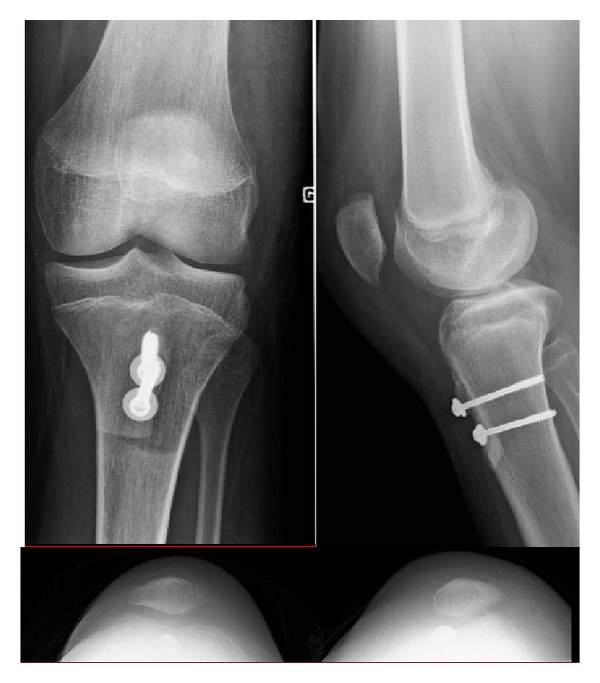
Postoperative radiograph of the knee after medial tibial tubercle transfer and vastus medialis plasty. The patella is better centred.
